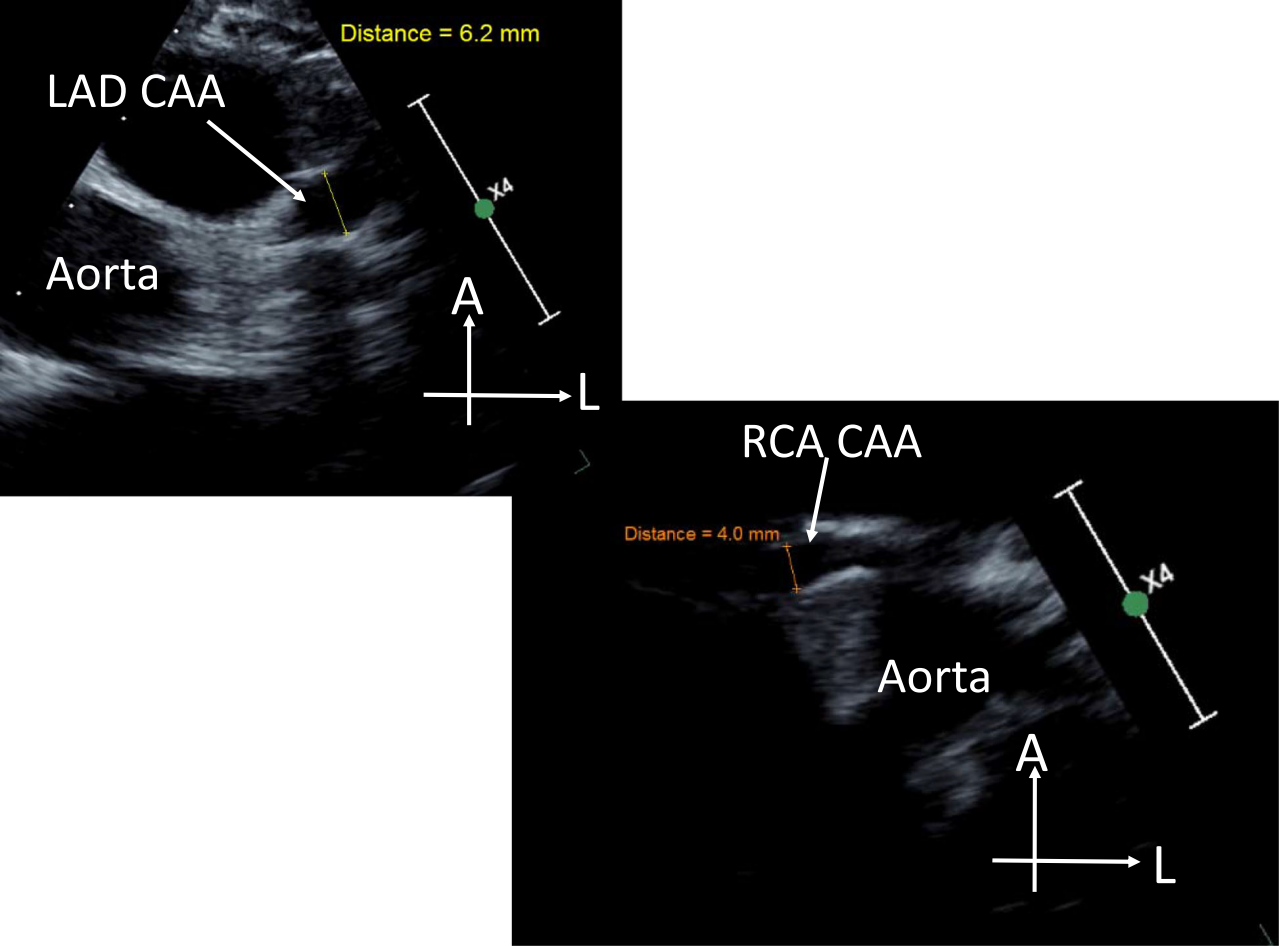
Echocardiogram images showing largest coronary artery aneurysms over follow-up. a Large/giant, saccular left anterior descending coronary artery aneurysm measuring ~ 6 mm. b Moderate-sized, fusiform proximal right coronary artery aneurysm. LAD, left anterior descending; CAA, coronary artery aneurysm; RCA, right coronary artery; A, anterior; L, left
